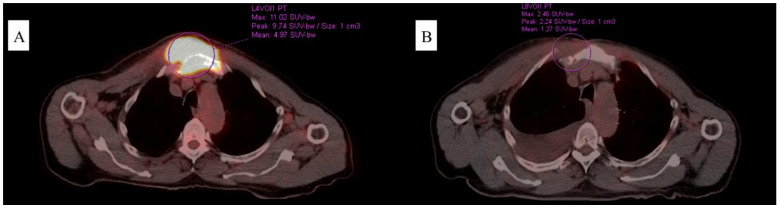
Comparison of the PET/CT scans upon diagnosis (A), and the best result achieved after treatment institution (B) related to Case 1.
